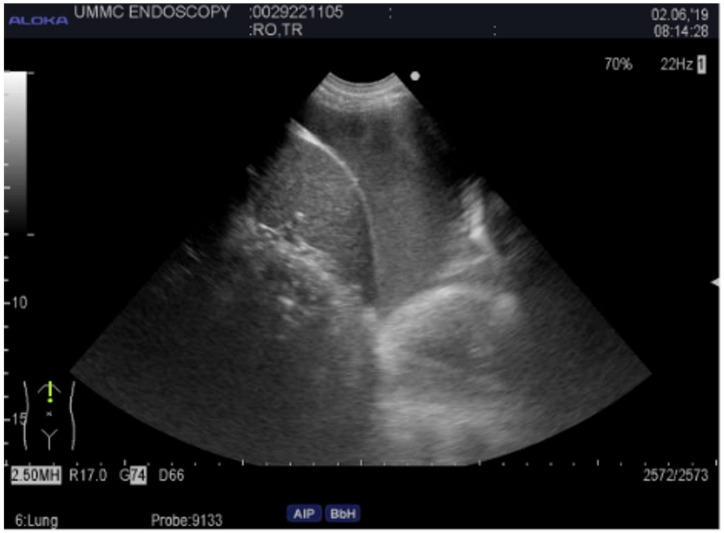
Ultrasound image of pleural effusion. Both thoracic and abdominal structures are seen, including the lung, diaphragm, and liver.
